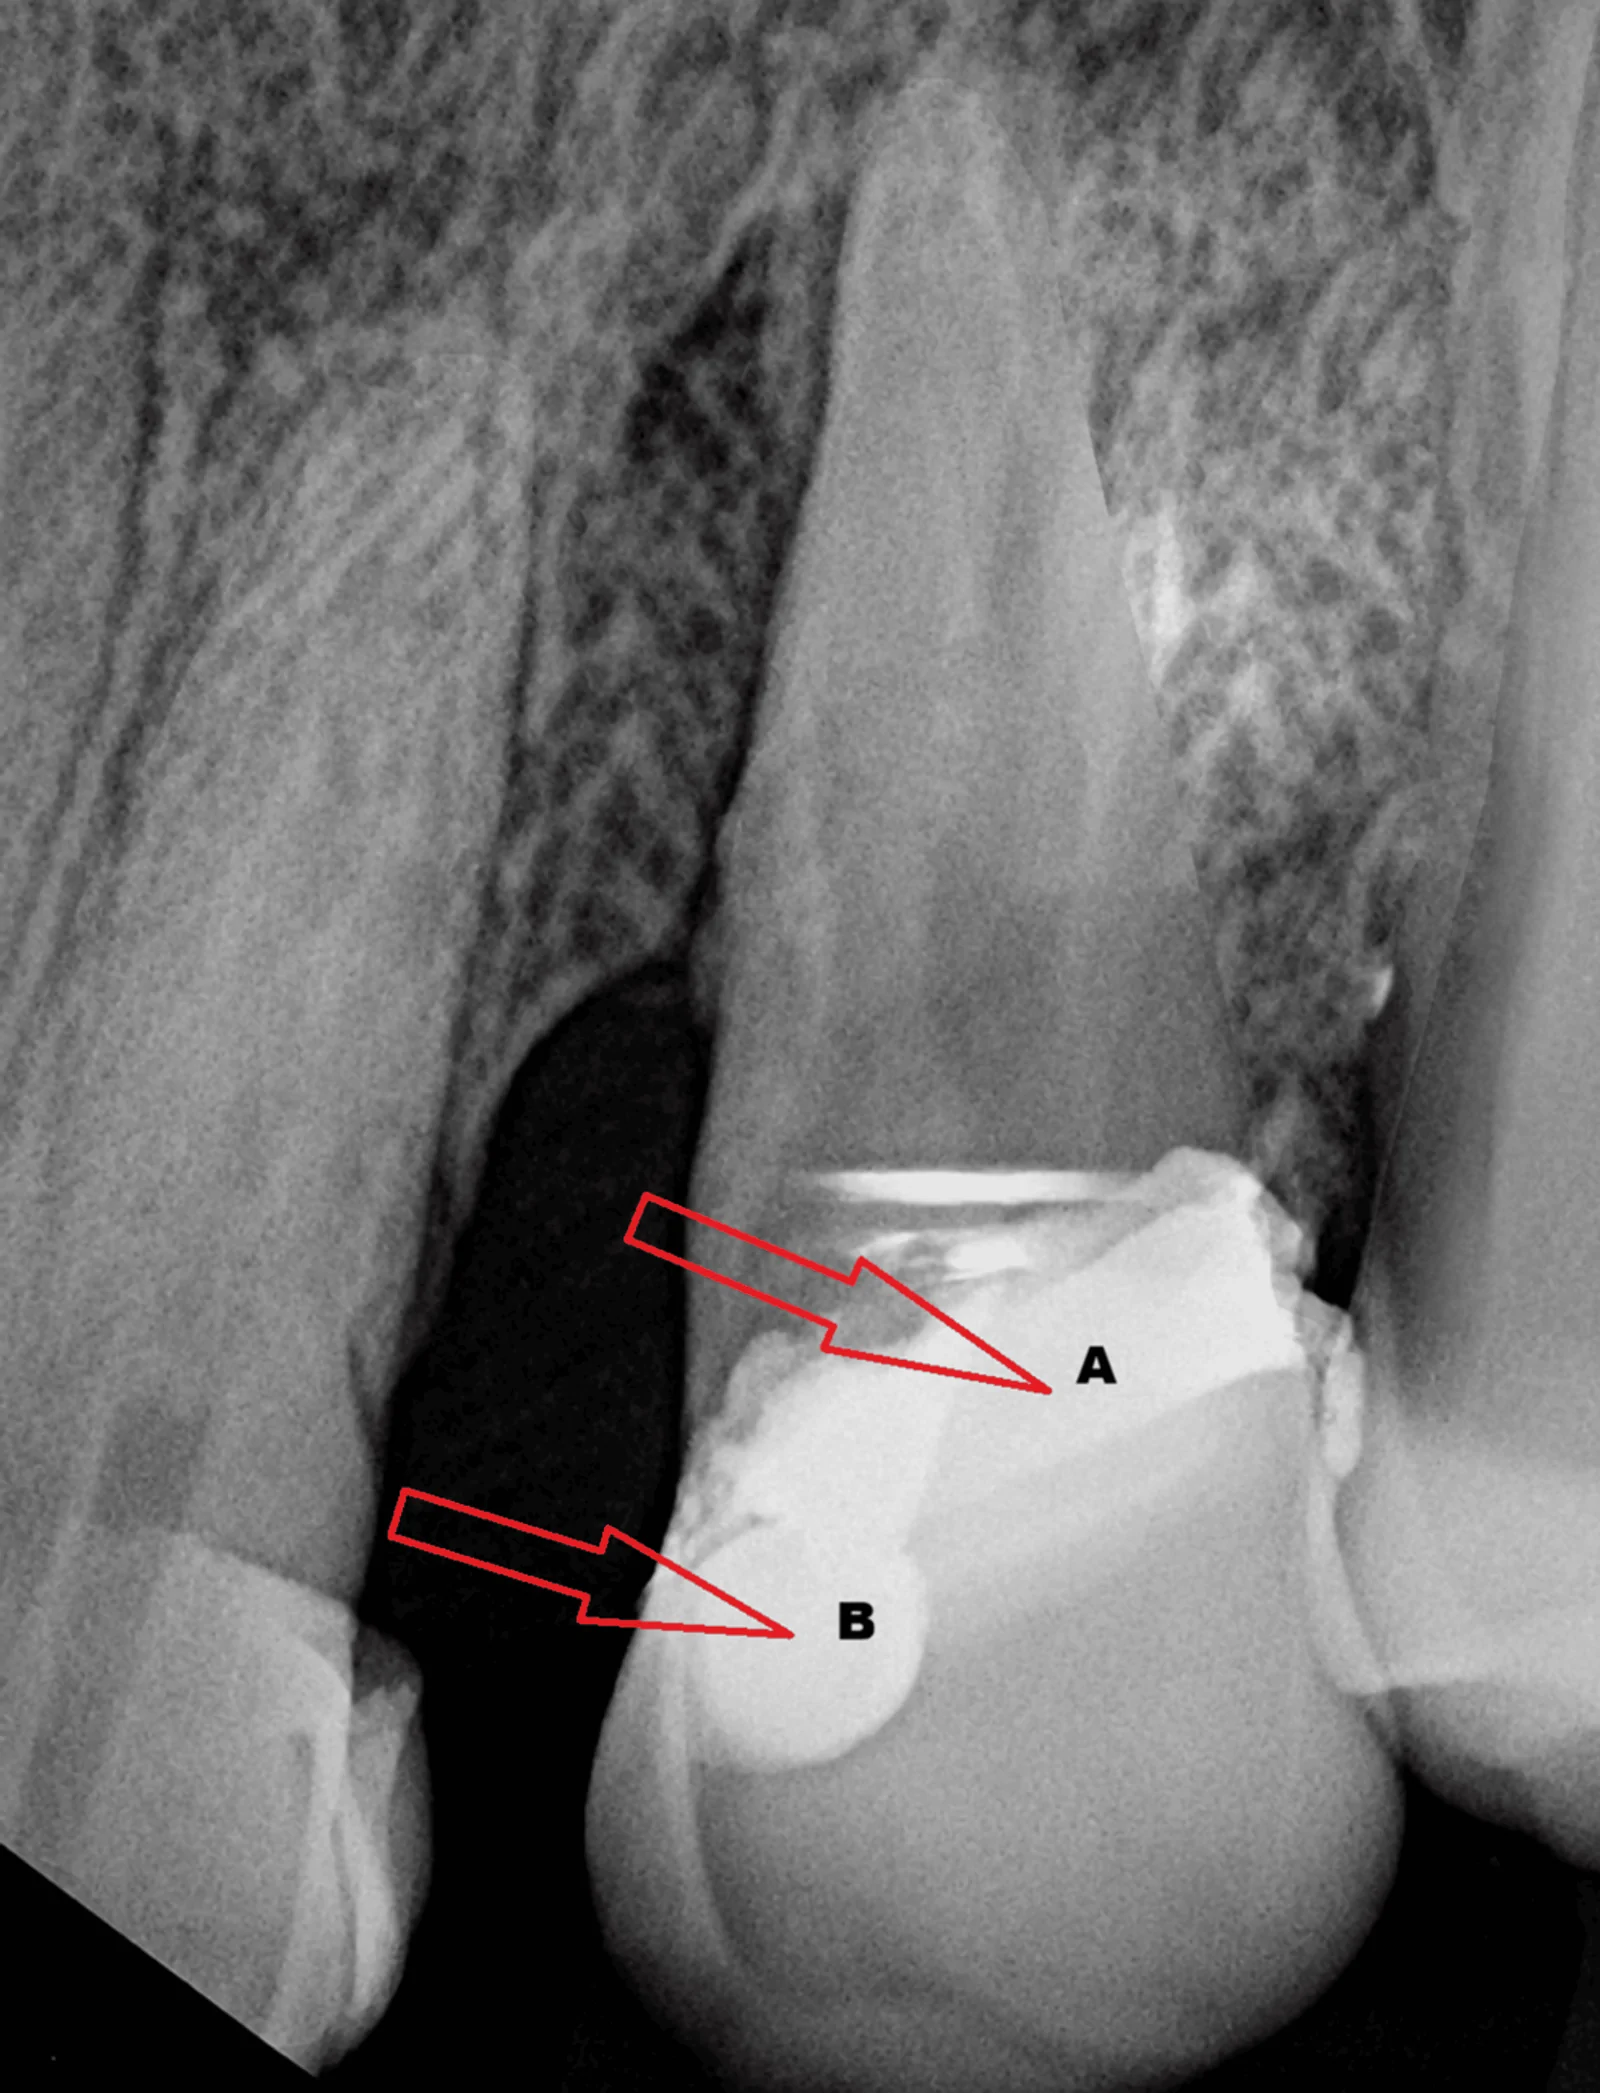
Preoperative Periapical Radiograph of Tooth #11. Preoperative radiograph demonstrating an extensive existing restoration (A) and limited remaining coronal tooth structure (B), resulting in an unfavorable restorative prognosis. Clinical examination also revealed increased tooth mobility associated with reduced periodontal support.
